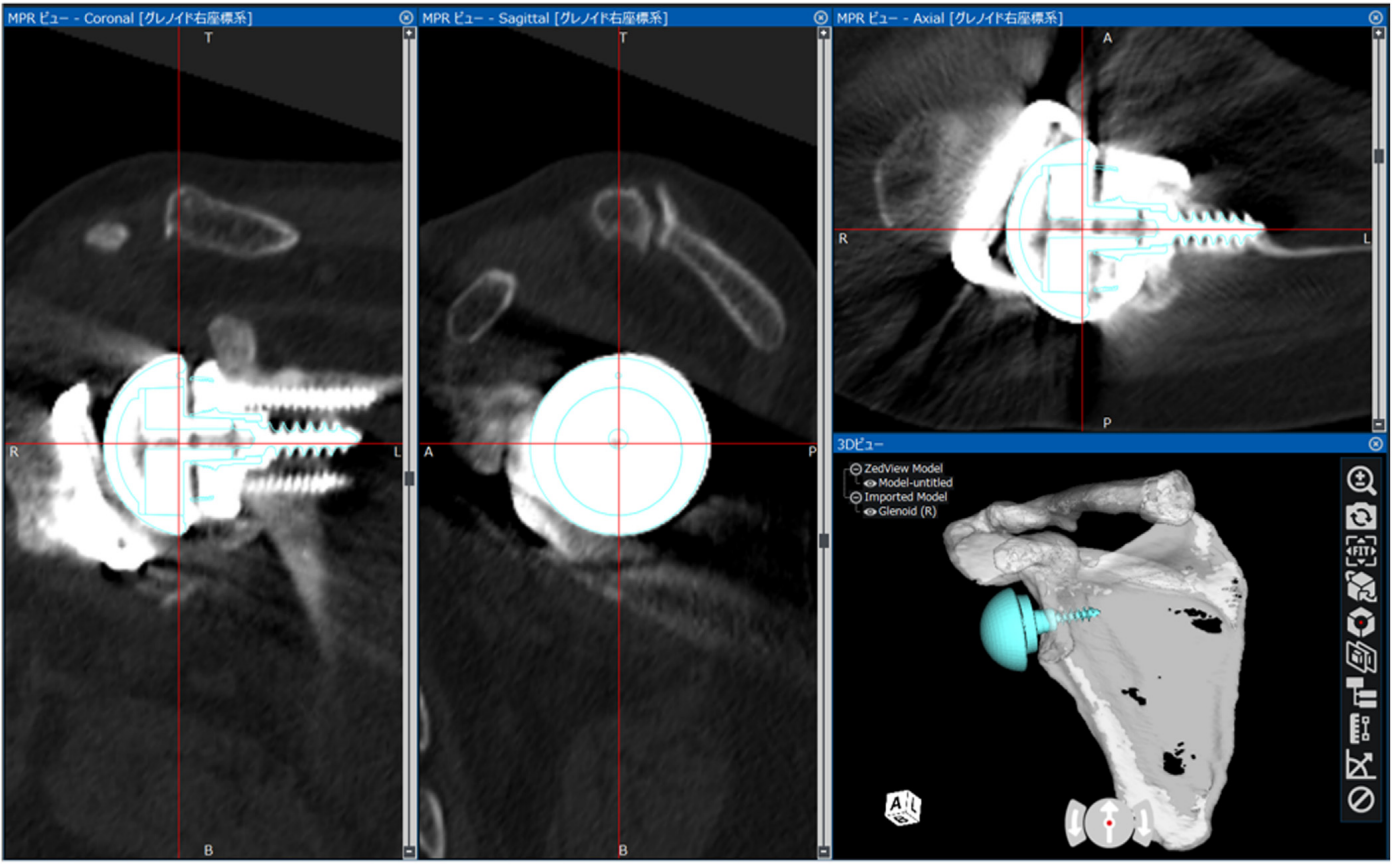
Implant matching method Zimmer Biomet's Comprehensive Reverse Shoulder System computer-aided design models were matched to the implants post-CT. *Red line*: line to create multiplanar reconstruction, *Blue line*: computer-aided design model of the Comprehensive Reverse Shoulder Arthroplasty System (Zimmer-Biomet, Warsaw, IN, USA). *CT*, computed tomography.
